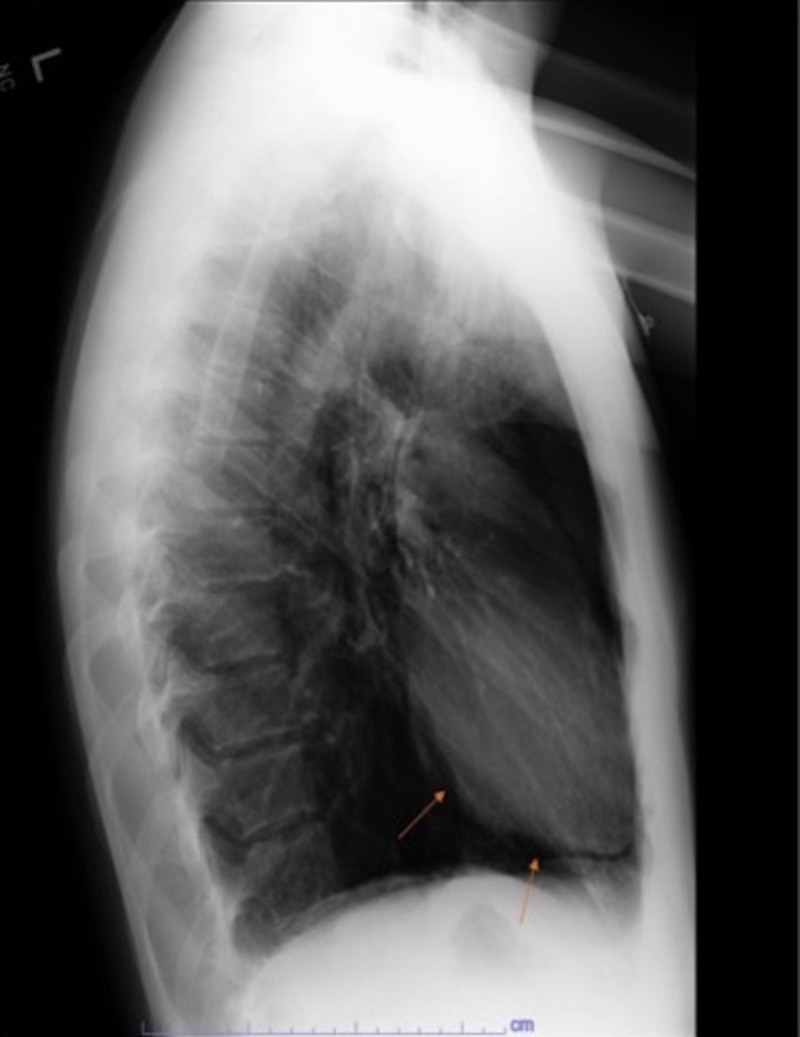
Lateral chest X-ray demonstrating pneumopericardium.
